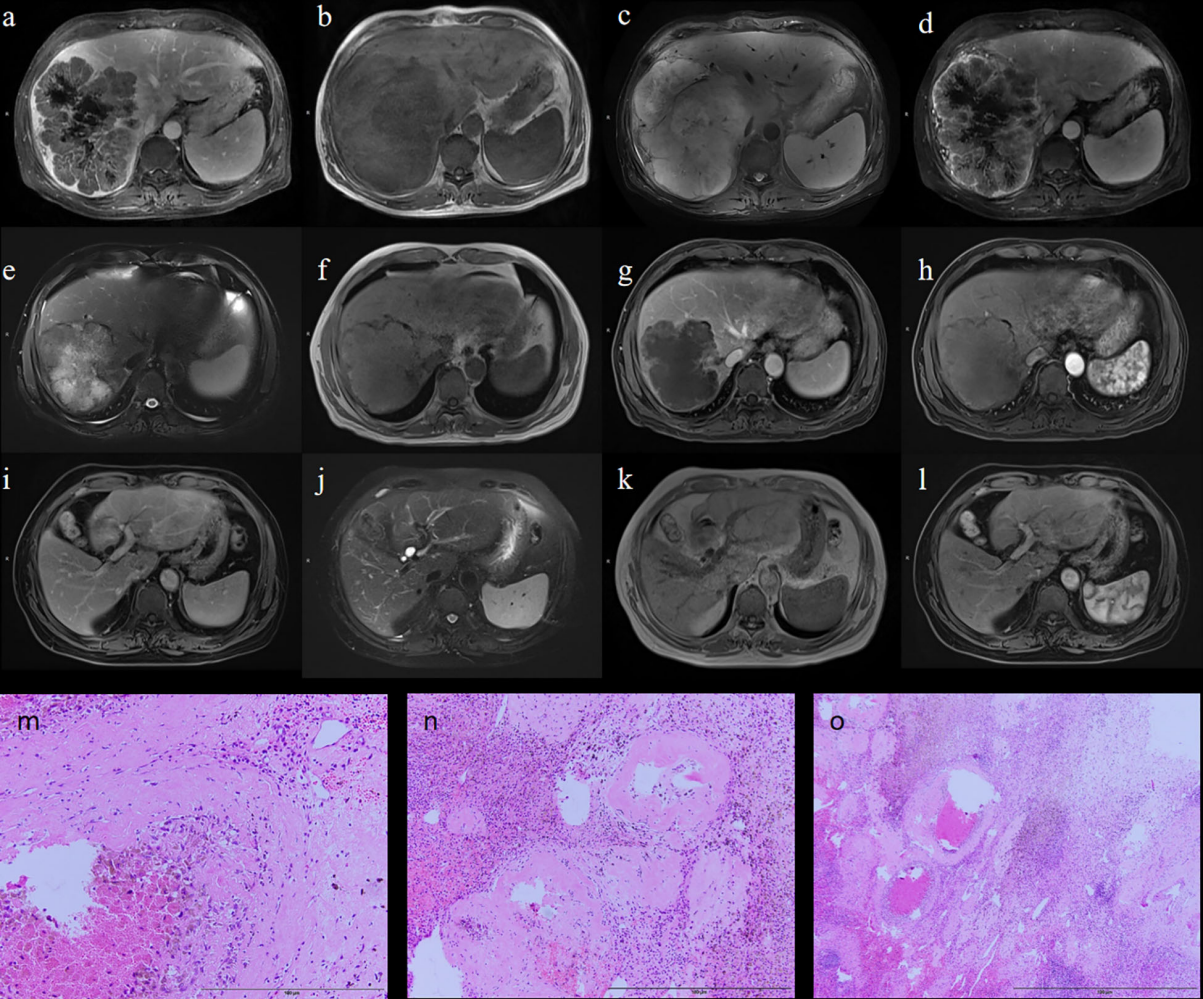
Representative case. Preoperative MRI (a–d); Postoperative MRI (e–h); MRI follow-up at 24 months after treatment (i–l); Intraoperative pathology (m–o).
